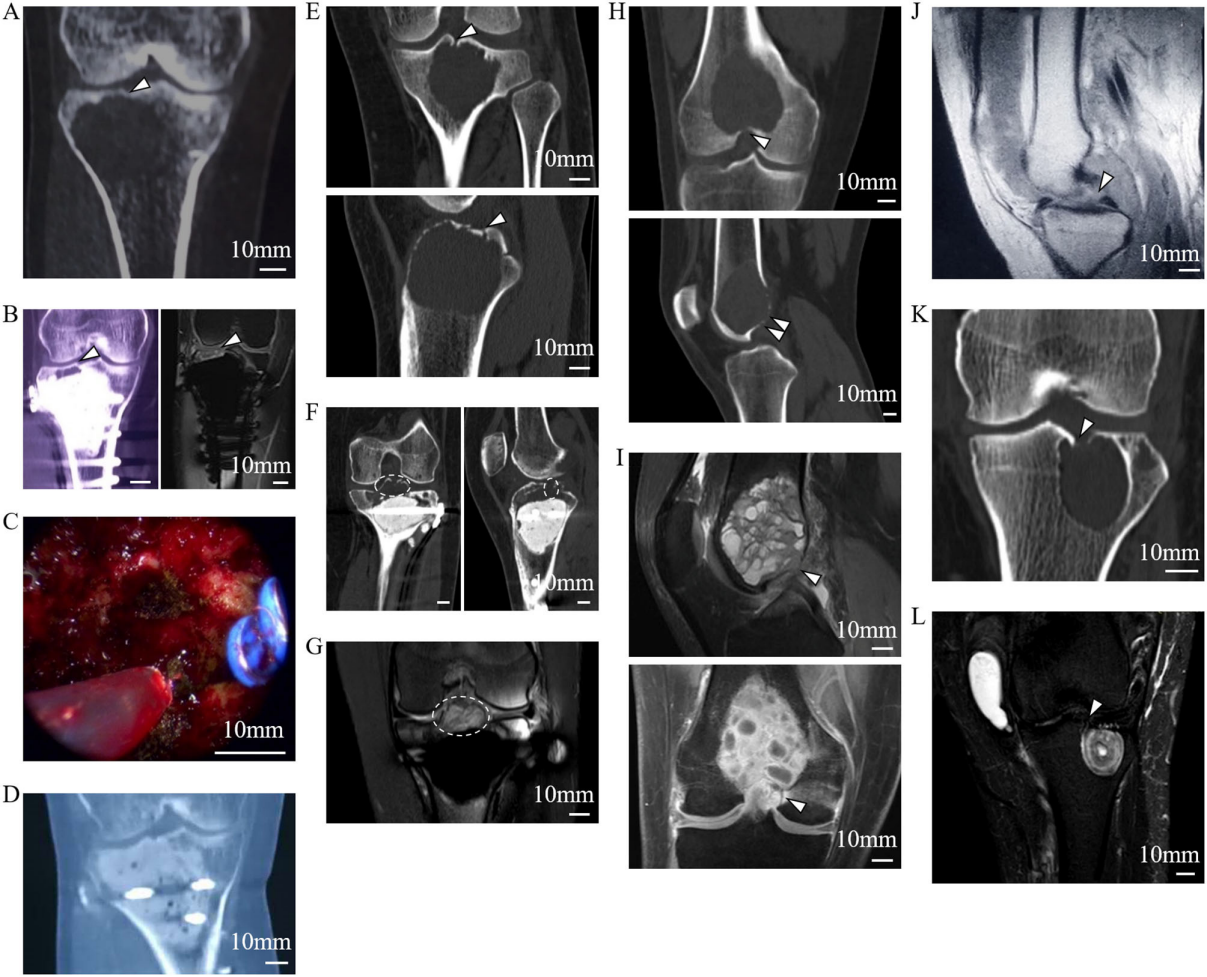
Tumor residue and extension in the LC complex on radiographic and intraoperative images. Images (A–D) from a 46-year-old female patient with recurrent GCTB. (A) Suspicious tumor residue shown in the tibial subchondral LC complex (arrowhead) in the first recurrence on CT. (B) Obvious GCTB recurrence shown in the tibial subchondral LC complex (arrowheads) on CT and MRI in the second recurrence. (C) LC complex ablation under arthroscopy. (D) No recurrence was observed for 24 months after LC complex ablation. Images (E–G) from a 20-year-old female patient with GCTB disseminating from the tibia to the articular cavity via the tibial LC complex. (E) Tumor invasion of the tibial LC complex (arrowheads) on the CT coronal and sagittal reconstruction before first operation. (F,G) The tumor recurred 13 months after curettage and extended into the articular cavity via the tibial LC complex (dash lines), as seen on CT (F) and MRI (G). Images (H,I) from a 24-year-old male patient with GCTB extension from the femur to the articular cavity via the femoral LC complexes (arrowheads) on CT (H) and MRI (I); the GCTB was confirmed following needle biopsy. Images (J–L) from a 52-year-old woman with recurrent diffused giant cell tumor of the tendon sheath; the intraarticular lesion disseminated to the tibia via the tibial LC complex. (J) D-TGCTS located above the tibial intercondylar region (arrowhead) on the MRI before synovectomy. (K) Osteolytic lesion occurred in the tibial LC complex (arrowhead) and intraosseous lesion shown on CT at 6 years after the synovectomy. (L) Tumor spread via the tibial LC complex (arrowhead) without damaging the peripheral cartilage on MRI.
